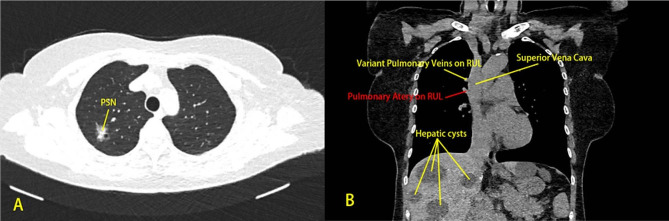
Pulmonary nodule on preoperative low-dose CT. PSN: Partial Solid Nodule, RUL: Right Upper Lobe
